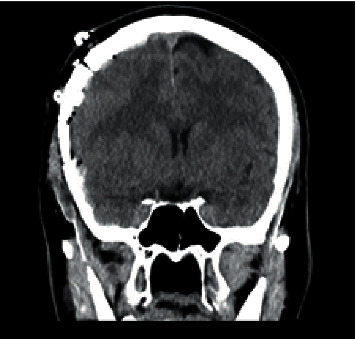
Noncontrast coronal CT head status after right frontoparietal craniotomy with subdural drain placement showing the evacuated right subdural hematoma with presence of residual-mixed density fluid and air.
